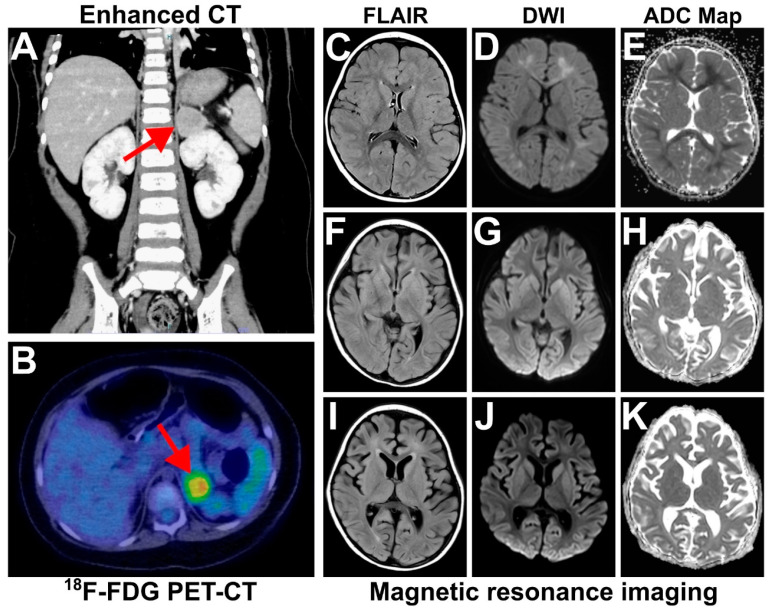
Main radiologic findings. (A) Enhanced computer tomography (CT) scan: coronal reconstruction. The arrow indicates the left adrenal tumor. (B) ^18^F-FDG positron transmission tomography–computer tomography (PET-CT) scan: axial slice. The arrow indicates high tracer uptake (SUVmax 4.69) in the left adrenal tumor. (C–K) Magnetic resonance images of the patient’s brain: (C–E) Day 1 (taken at the previous hospital), (F–H) Day 15 (before treatment), (I–K) Day 37 (after treatment).
